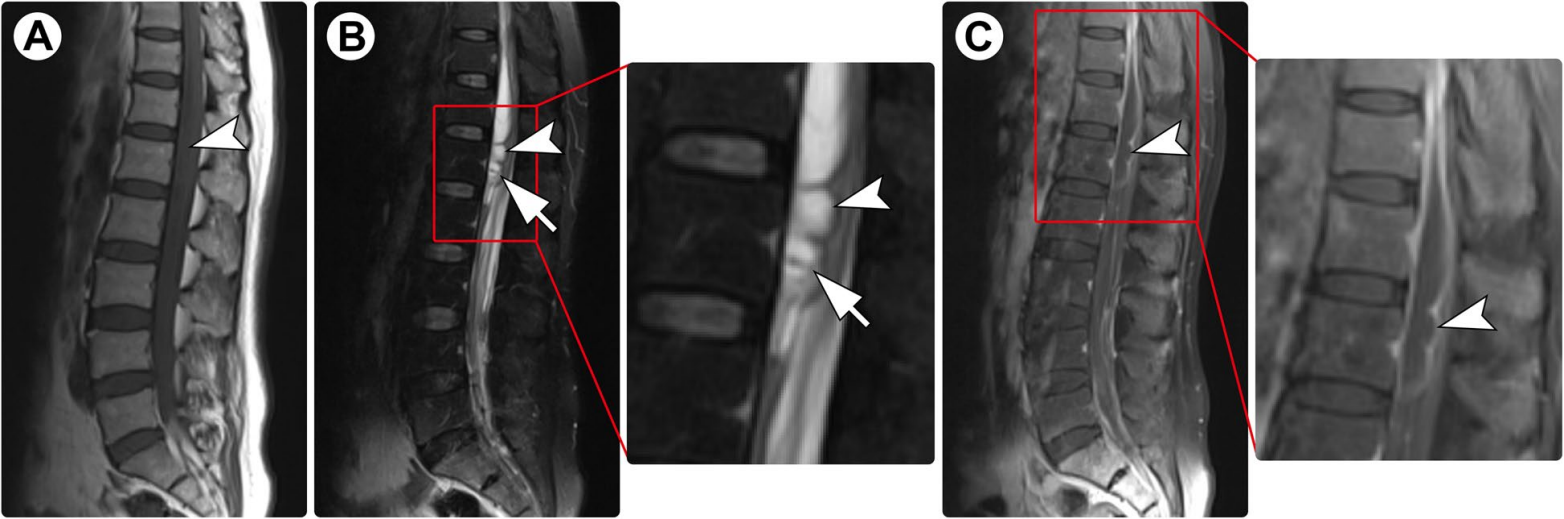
Spinal MRI of Case 7. Spinal MRI showed a septated cyst (arrowheads) at the T11-T1 levels, appearing slightly hypointensity on T1-weighted imaging (A) and hyperintensity on T2-weighted imaging (B). Additionally, an isointense mural nodule (arrows) was noted on T2-weighted imaging (B). After administration of contrast medium, cyst wall enhancement was demonstrated (C)
